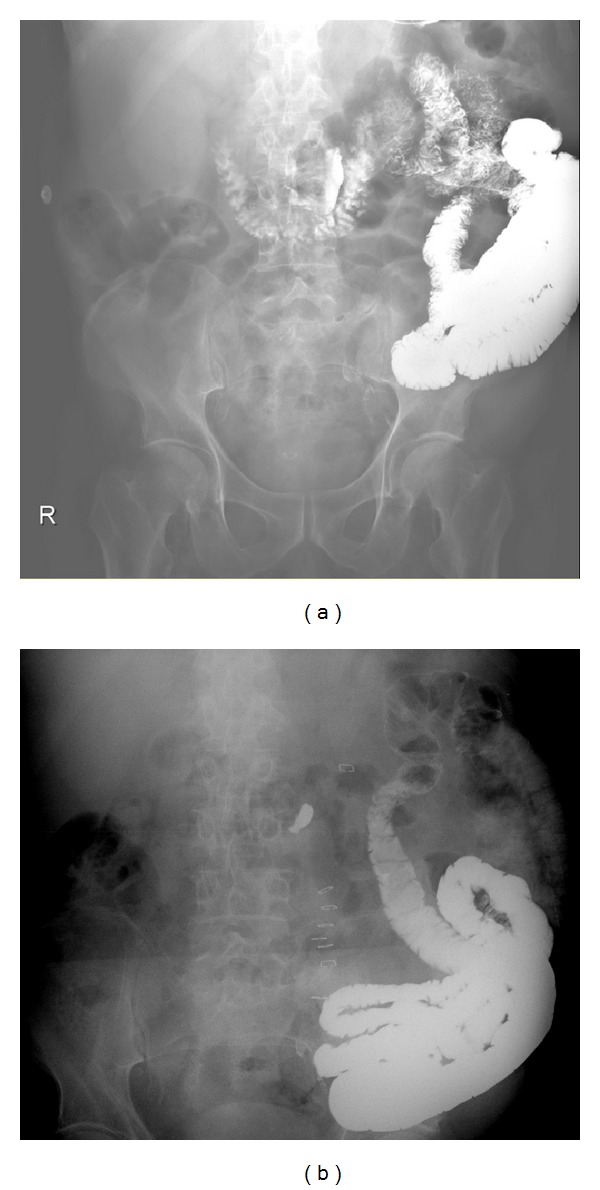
(a) Abdominal X-ray taken three days before the patient's small bowel resection. (b) Abdominal X-ray taken nine days after the patient's small bowel resection. Note how the contrast has not progressed and remains in the distal jejunum and ileum.
